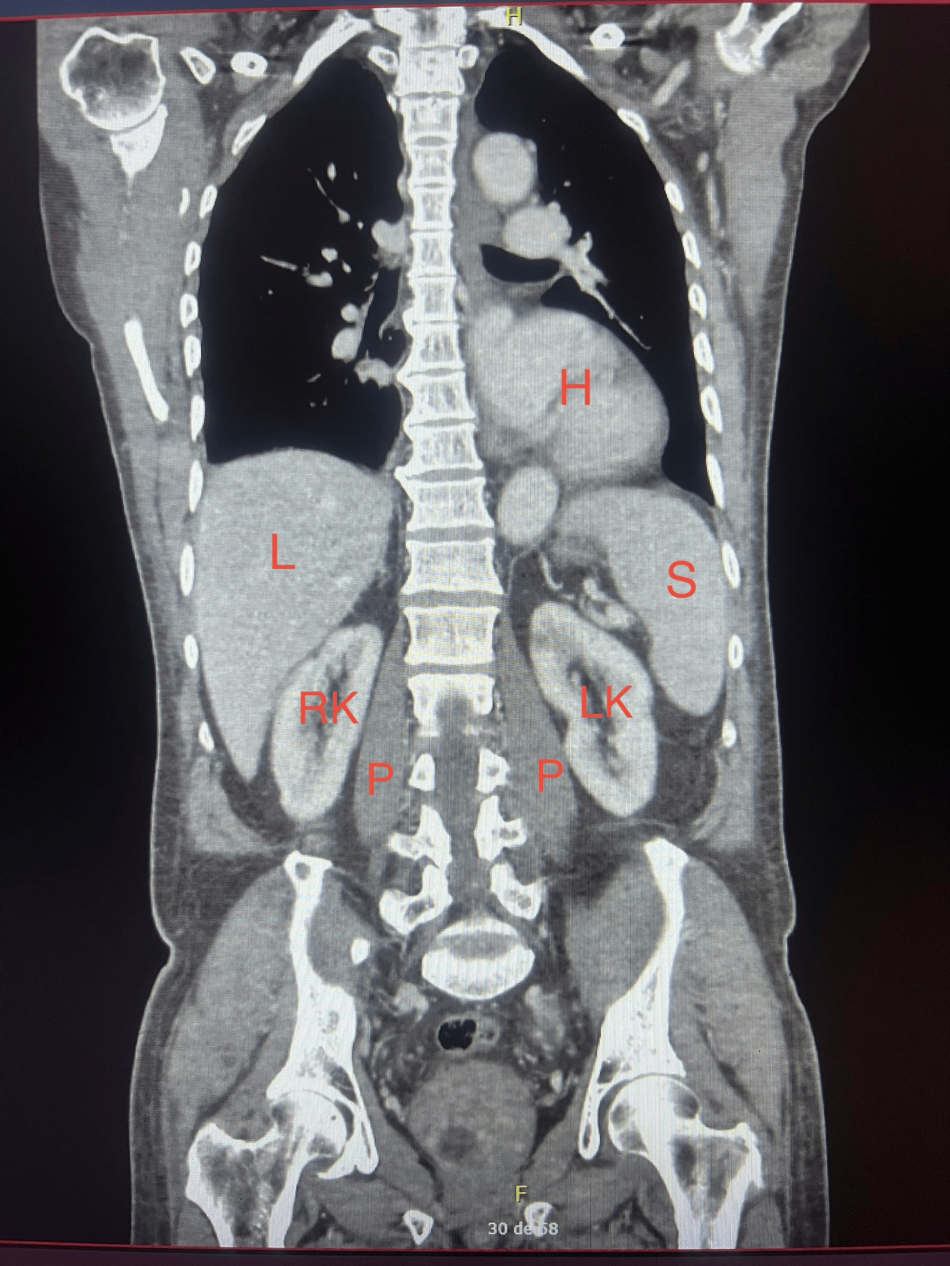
Coronal multiplanar reconstruction of contrast-enhanced abdominopelvic CT (retroperitoneal view) Coronal multiplanar reconstruction of contrast-enhanced abdominopelvic CT (portal venous phase), demonstrating absence of intra-abdominal or pelvic fluid collections or abscess formation on the retroperitoneum. H=Heart, =Liver, S=Spleen, RK=Right kidney, LK=Left kidney, P=Psoas muscle. Informed consent was given for the publication of the study.
